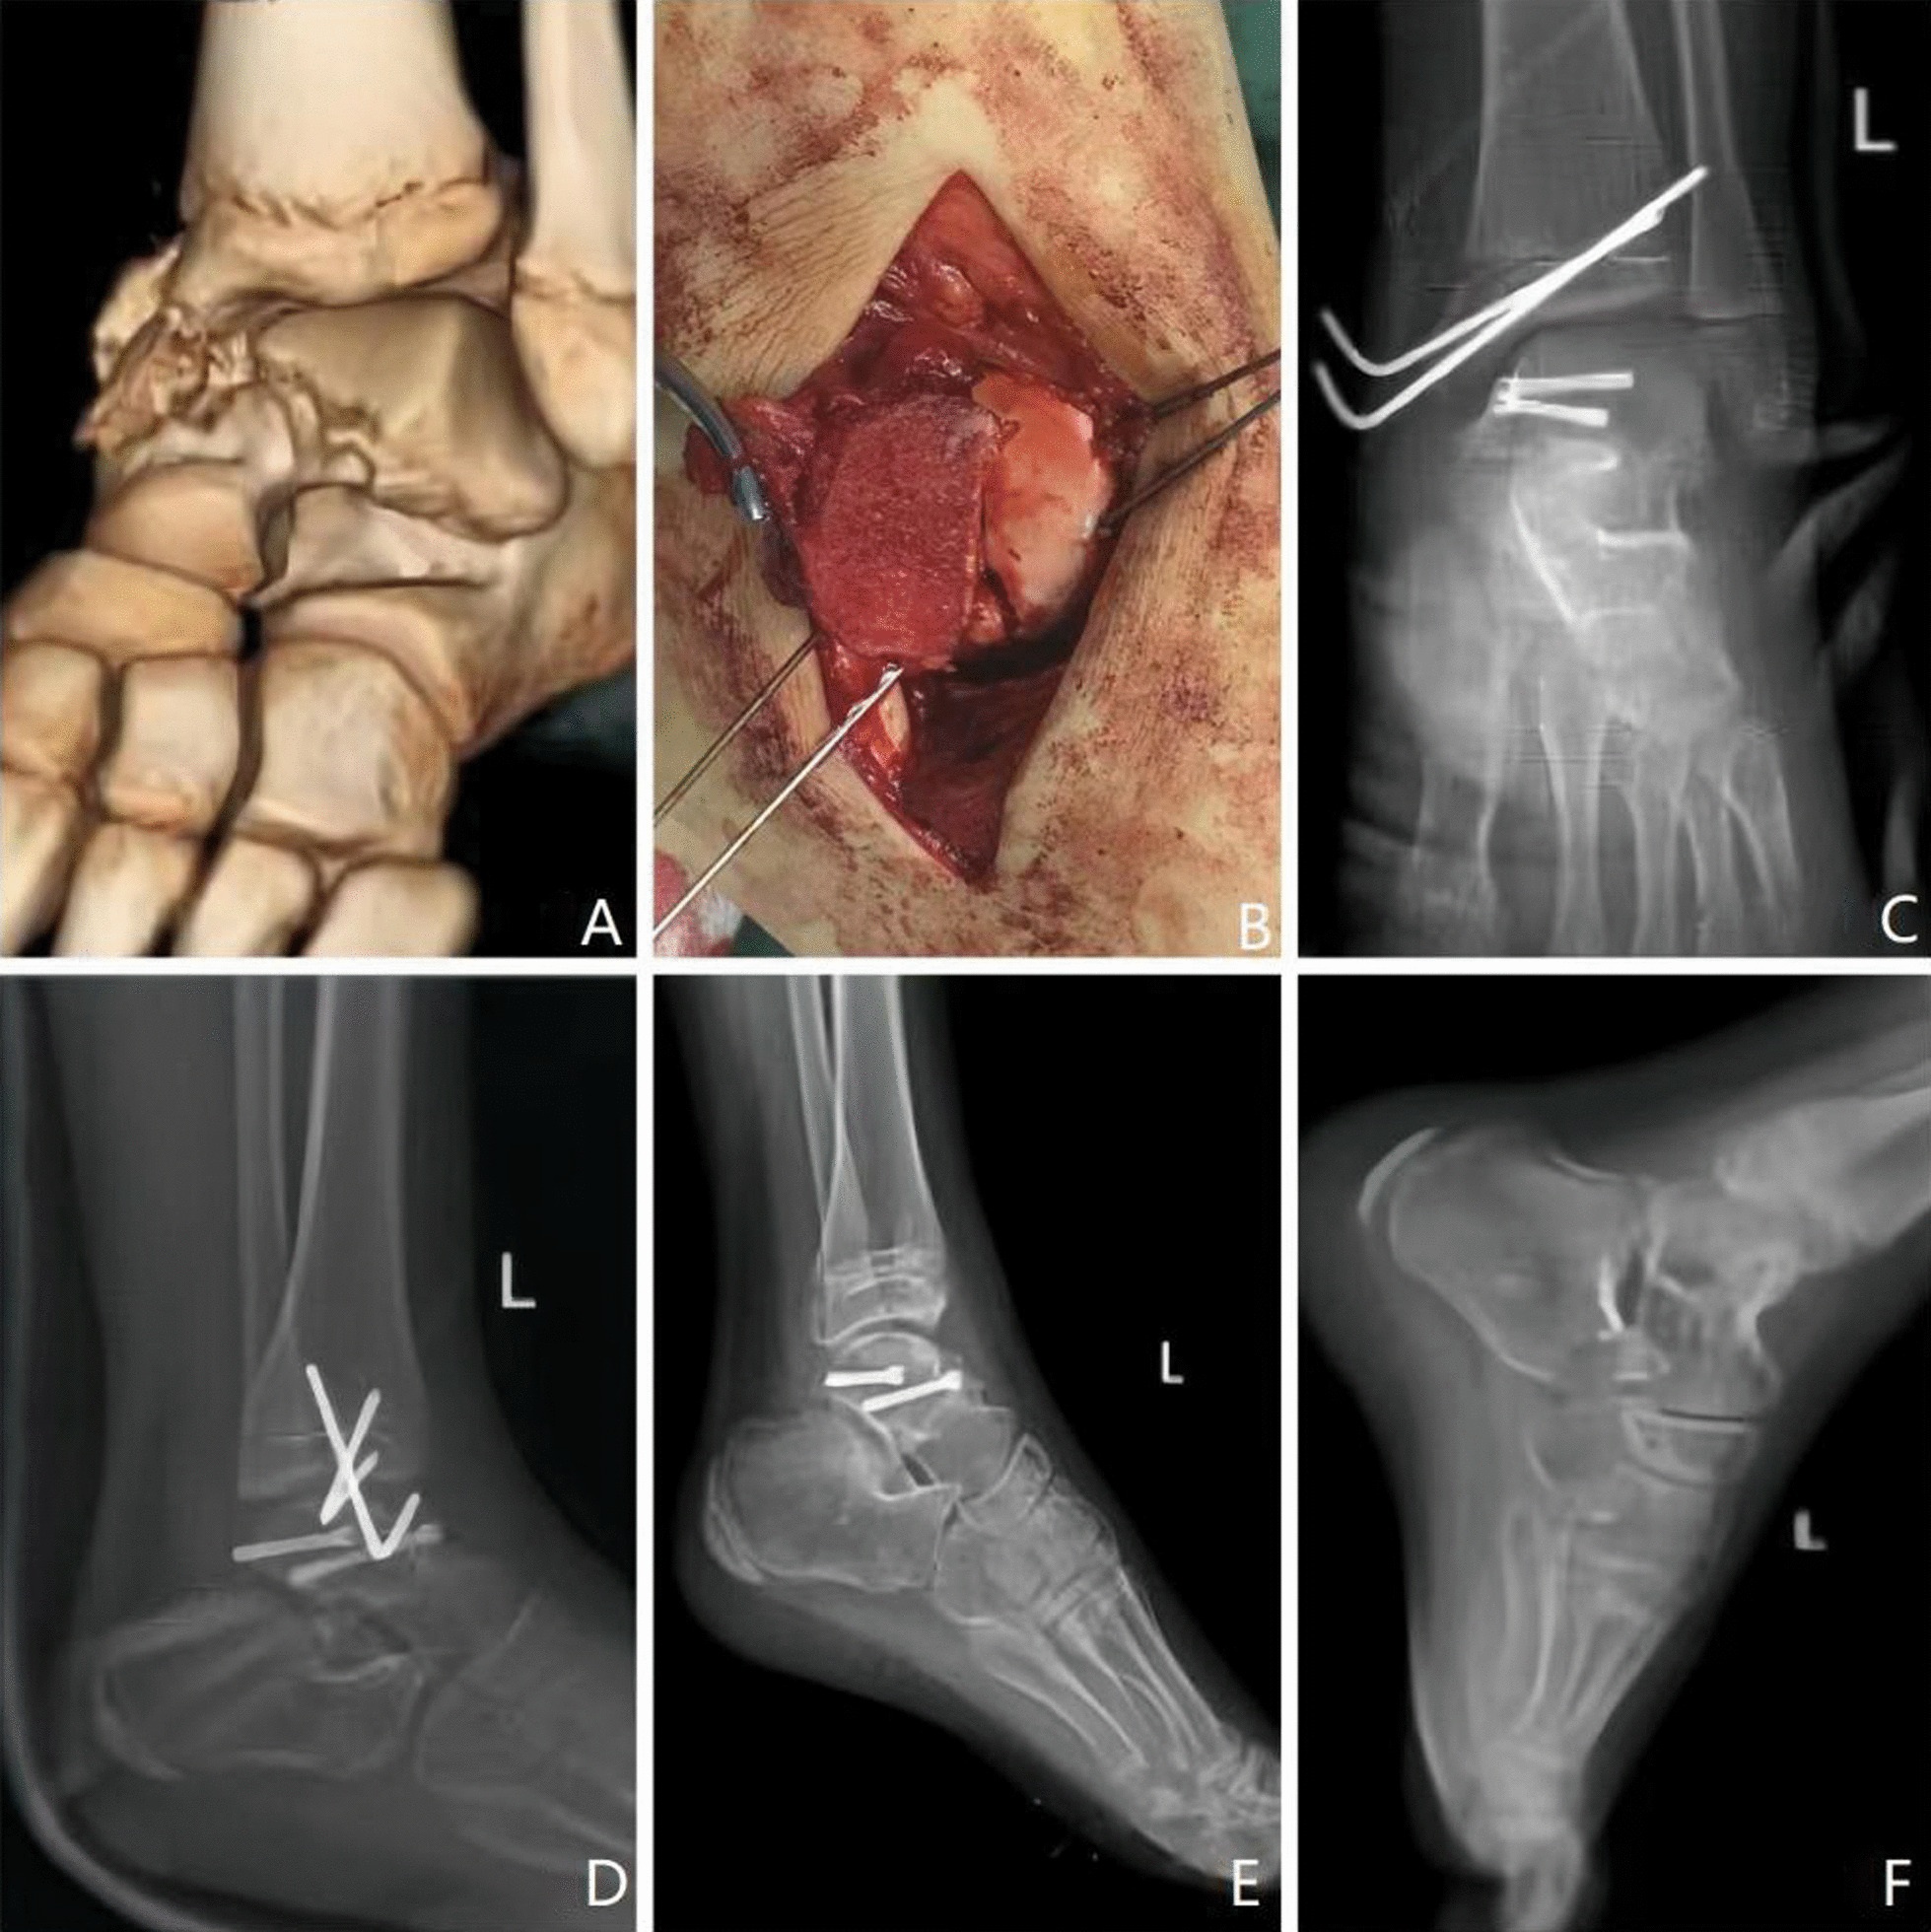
A 7-year-old girl suffered from a left talar fracture caused by a traffic accident. The preoperative CT (A) and intraoperative (B) images showed a type II fracture of the left talus neck. Postoperative X-ray of the ankle joint (C, D) showed good reduction in the fracture. At 6 months after the operation, anteroposterior and lateral X-ray films of the ankle joint (E) showed that the fracture had healed, and the internal fixation was removed (F). At 48 months after the operation, the adduction function of the left ankle joint was worse than that of the contralateral side, and the plantar flexion and dorsiflexion functions were normal. The AOFAS score was 93
